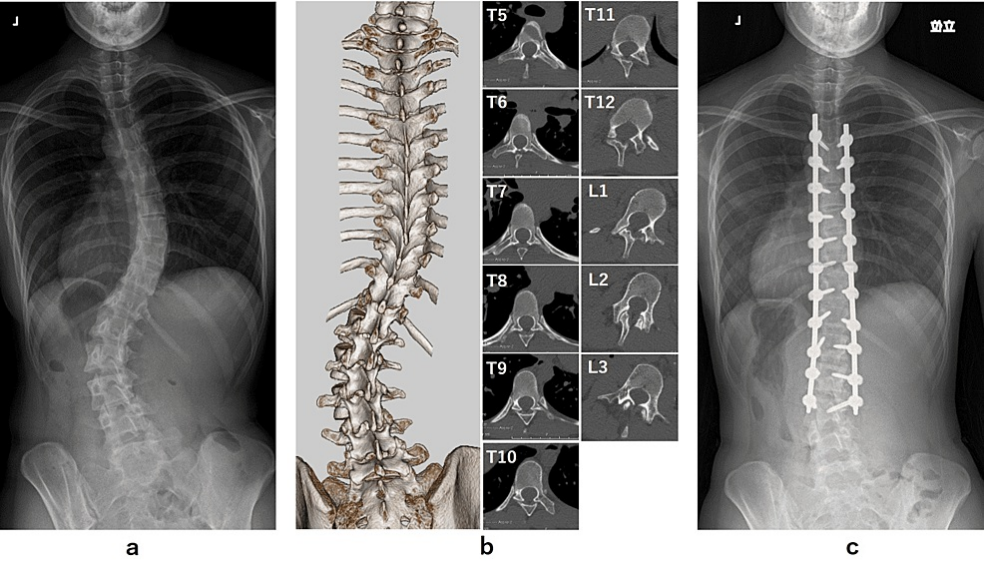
The imaging data of the patients with adolescent idiopathic scoliosis. a. Preoperative standing radiograph b. Preoperative computed tomography c. Postoperative standing radiograph
